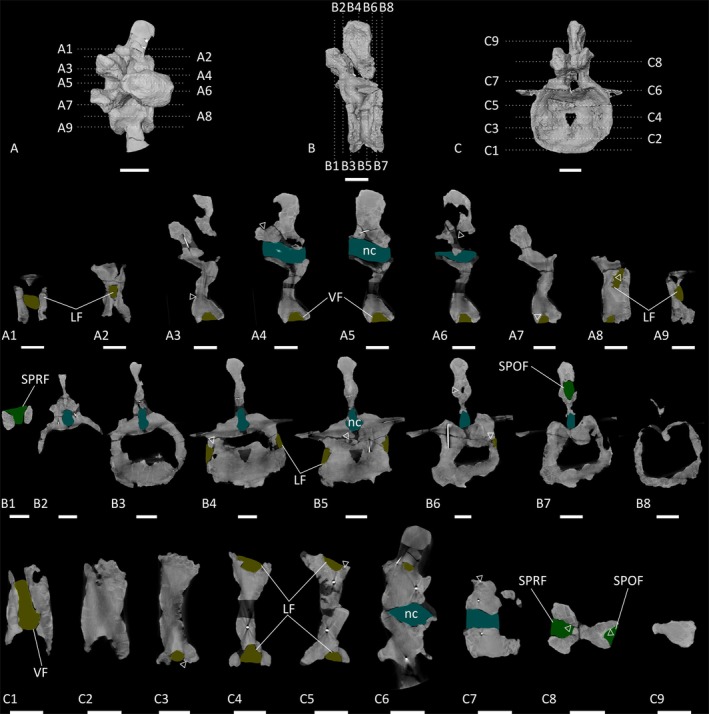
CT scan of *Giraffatitan brancai* caudal vertebra 2 MB.R.5000.1. (A–C) 3D rendering (A) dorsal view, (B) left lateral view, (C) anterior view; (A1–A9) CT scan slices in parasagittal cross‐section, (B1–B8) CT scan slices in transverse cross‐section, (C1–C9) CT scan slices in horizontal cross‐section. LF, lateral fossa; nc, neural canal; SPOF, spinopostzygapophyseal fossa; SPRF, spinoprezygapophyseal fossa; VF, ventral fossa. Key: Blue highlight, neural canal; green highlight, neural spine fossa; yellow highlight, centrum fossa; white arrow, pneumatic bone texture. Scale bars = 100 mm.
